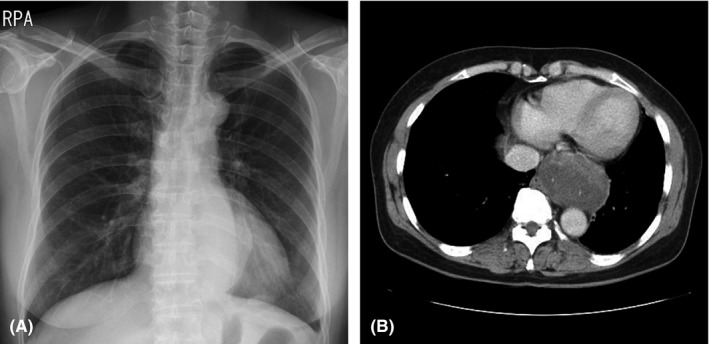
Chest X‐ray showed mediastinal mass in posterior cardia area (A). The chest computed tomography showed an oval shaped mass of 7.4 × 4.5 cm size in the posterior mediastinum. The mass compressed the left atrium, esophagus, and no definite invasion to lung (B)
